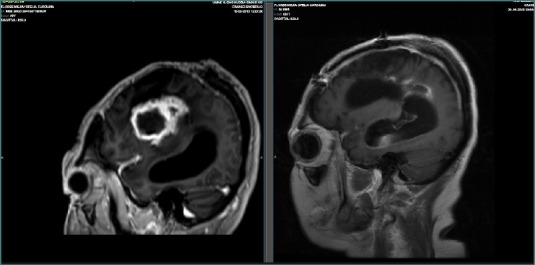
Sagittal MRI images before and after multimodal treatment
